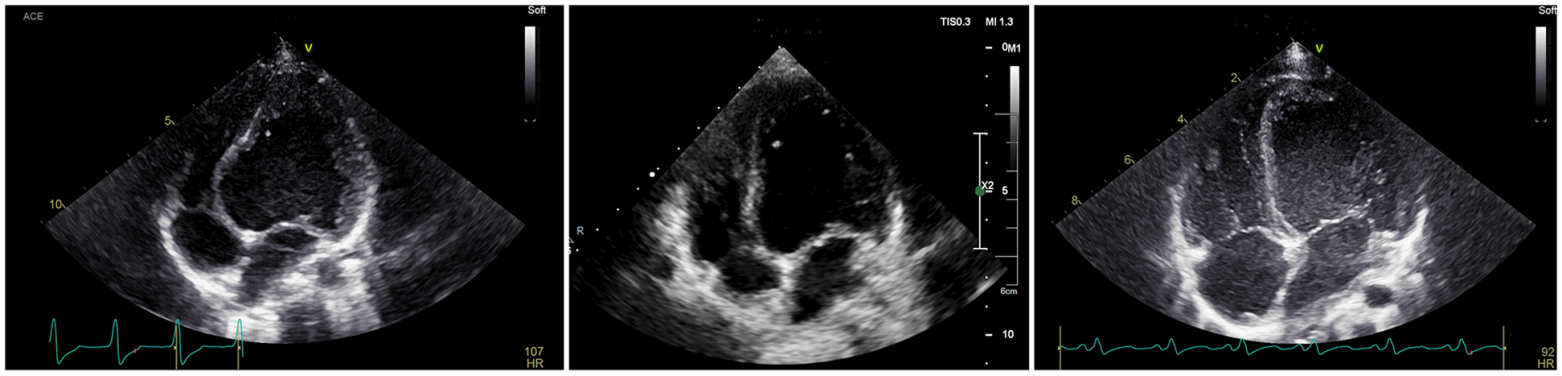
The patient, male, 8 years old, was admitted to the hospital for RFA treatment for WPWs, and intraoperative diagnosis of type B bypass was made. Preoperatively, the LV of the parasternal four-chambered heart was globularly dilated, and the septum was significantly convex towards the right ventricle; on review 1 week after the operation, the LV was significantly smaller than before the operation, and the base of the septum was slightly convex towards the right ventricle; on review 3 month after the operation, the four-chambered heart showed that the left and right hearts were in good proportion to each other.
